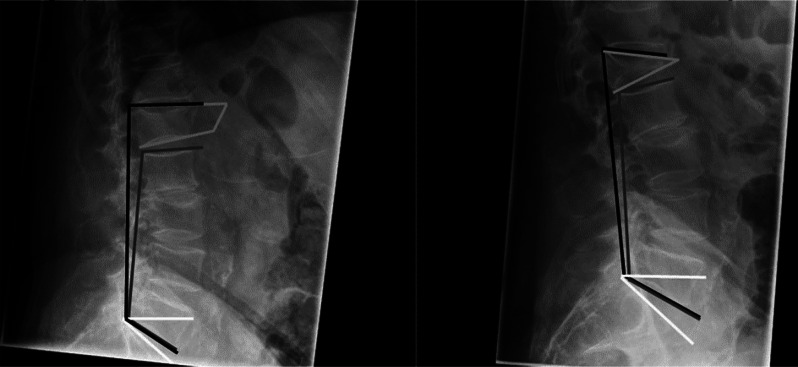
Exemplary measurements in lateral radiographs. Lateral radiographs demonstrate plotted measurements of the following spinal parameters: total lumbar lordosis (black), lordosis caudal to the fracture (dark grey), kyphosis of the fractured vertebra (light gray) and sacral slope (white)
